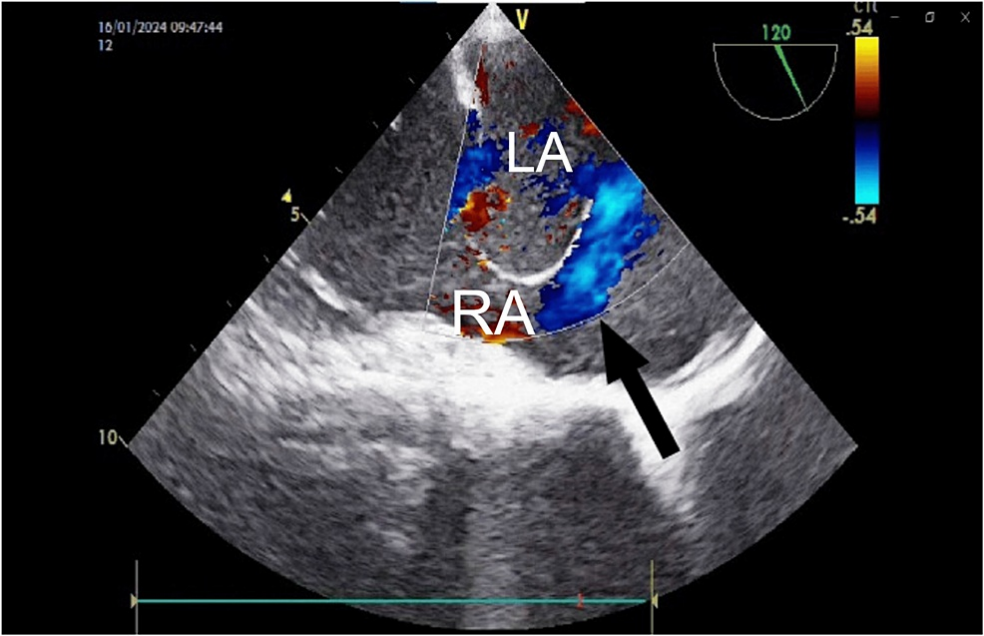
Transesophageal echocardiographic (TEE) image showing shunting across the atrial septal defect (black arrow). RA: right atrium; LA: left atrium.
